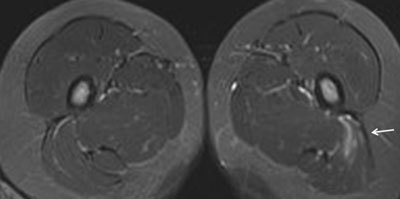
Axial STIR MR image in an 8-year-old girl with enthesitis-related arthritis shows enthesitis of the left gluteus maximus insertion (arrow).
